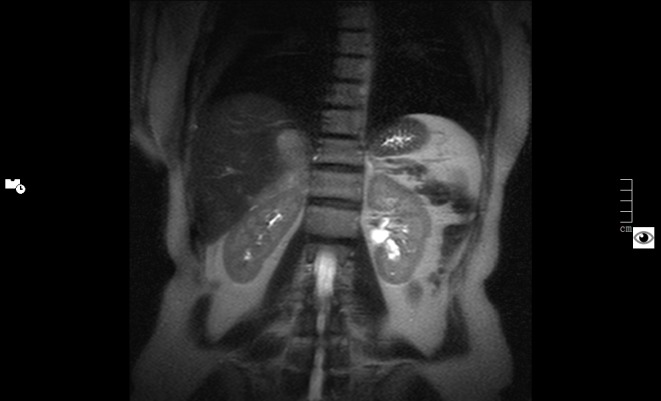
Metastatic spread of pheochromocytoma in right liver lobe, right kidney, and right hemidiaphragm in abdominal MRI
